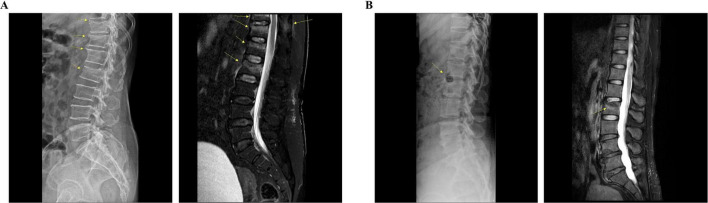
Radiological findings of each patient. (A) Patient 1: lateral radiograph reveals subtle bone deformities, including radiolucent lines, extending from T11 to L2. Sagittal T2-weighted fat-saturated MRI shows low signal intensity lines with bone marrow edema in the T12, L1, and L2 vertebral bodies, indicative of recent fractures. Additionally, a recent fracture can be observed at the T11 vertebral body, accompanied by bone marrow edema in the spinous process. (B) Patient 2: lateral radiograph reveals bone lesions, including a hypo-dense lesion in the anterior column of the L2 vertebra. Sagittal T2-weighted fat-saturated MRI demonstrates a compression fracture with bone marrow edema in the L2 vertebral body. MRI, magnetic resonance imaging.
